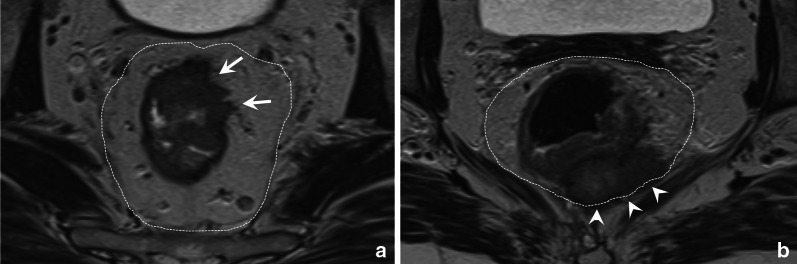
Axial T2-weighted images of a low risk T3ab tumour without MRF involvement (a) and a high-risk T3cd tumour with MRF involvement (b). The left patient is a 52 year old male patient with a tumour that extends beyond the muscularis propria from approximately 12 till 2 o’clock (white arrows) with an extramural invasion depth of < 5 mm. There is a sufficient margin (> 1 mm) between the tumour and MRF. The right patient is a 55-year-old female patient with extensive extramural invasion from 4 to 6 o’clock with broad-based involvement of the MRF (white arrowheads)
